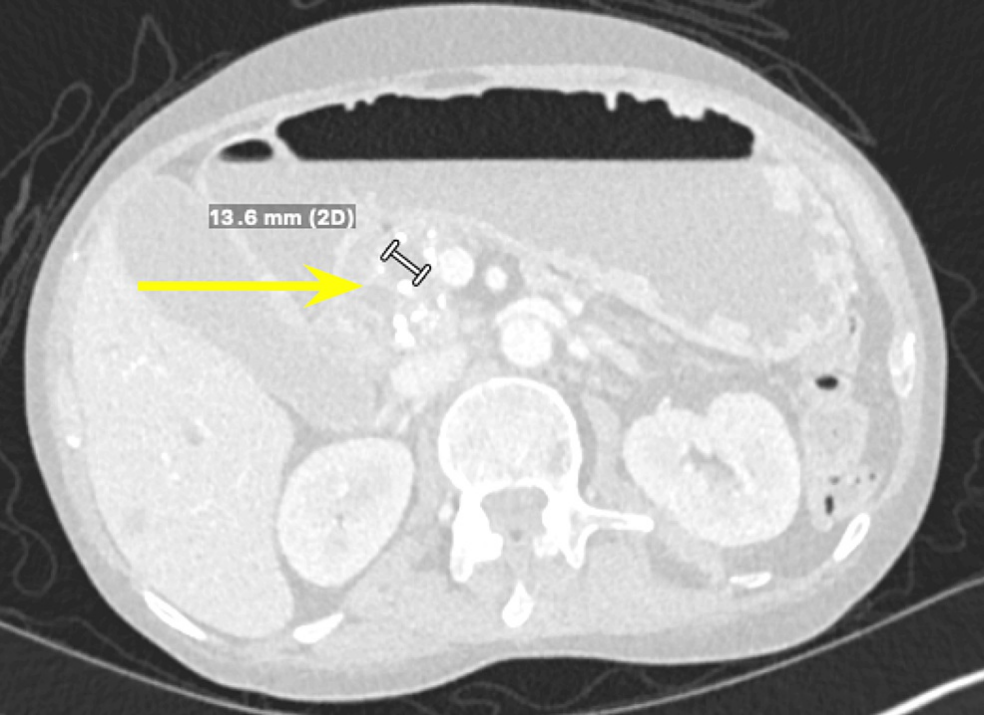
Computed tomography of the abdomen and pelvis showing a hepatic lesion, measuring 1.36 cm.
